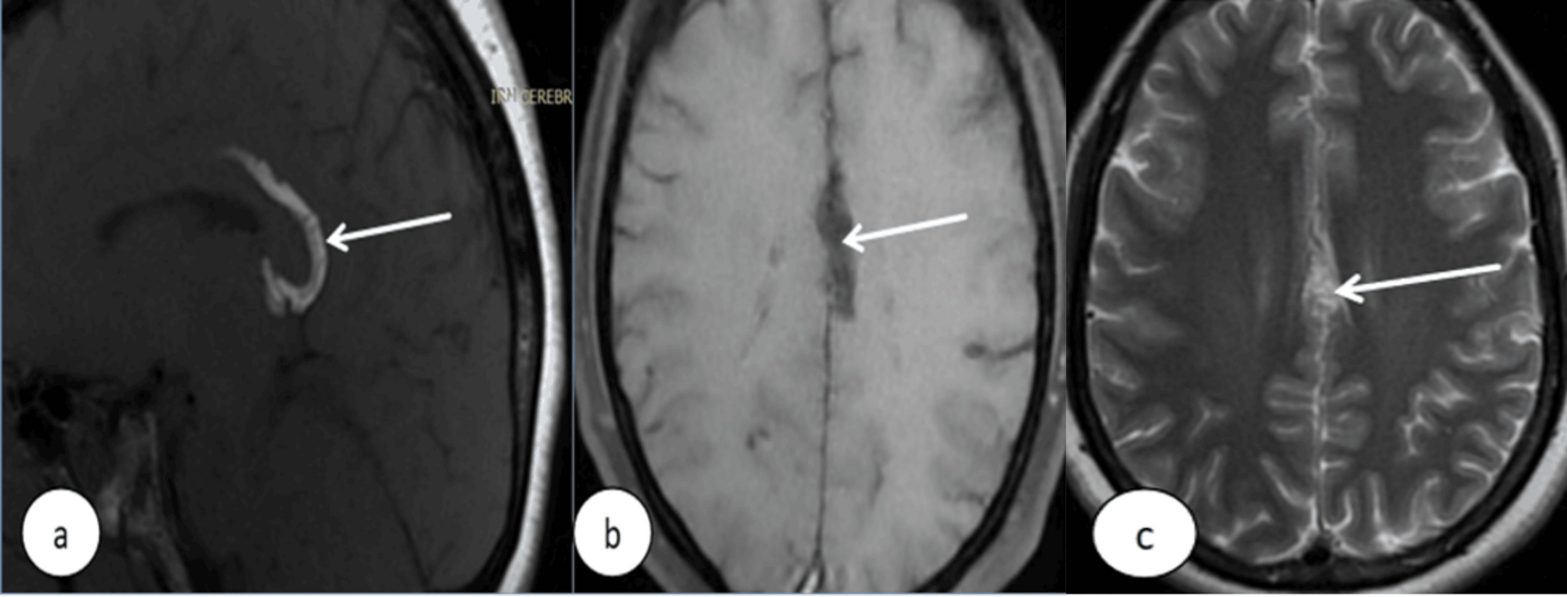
Brain MRI with (a) sagittal T1 FSE, (b) axial T1 Fat Saturation, and (c) axial T2 showing a curvilinear pericallosal lipoma (arrows), appearing hyperintense on T1 and T2, with signal loss on the T1 Fat Saturation sequence. MRI: magnetic resonance imaging (MRI); T1 FSE: fast spin echo; T1 Fat Sat: MRI T1 fat saturated
